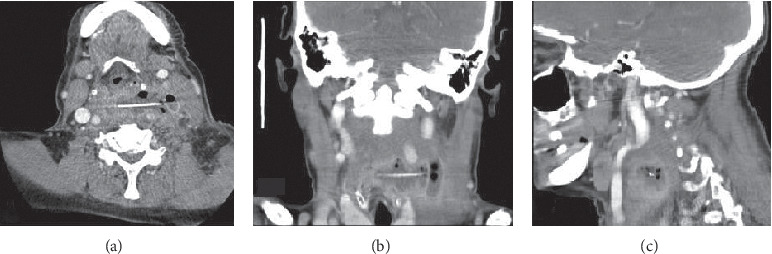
Fishbone foreign body revealed in CT scan. (a) Axial view, (b) coronal view, and (c) sagittal view of the foreign body with horizontal orientation in the superior part of the pharynx.
